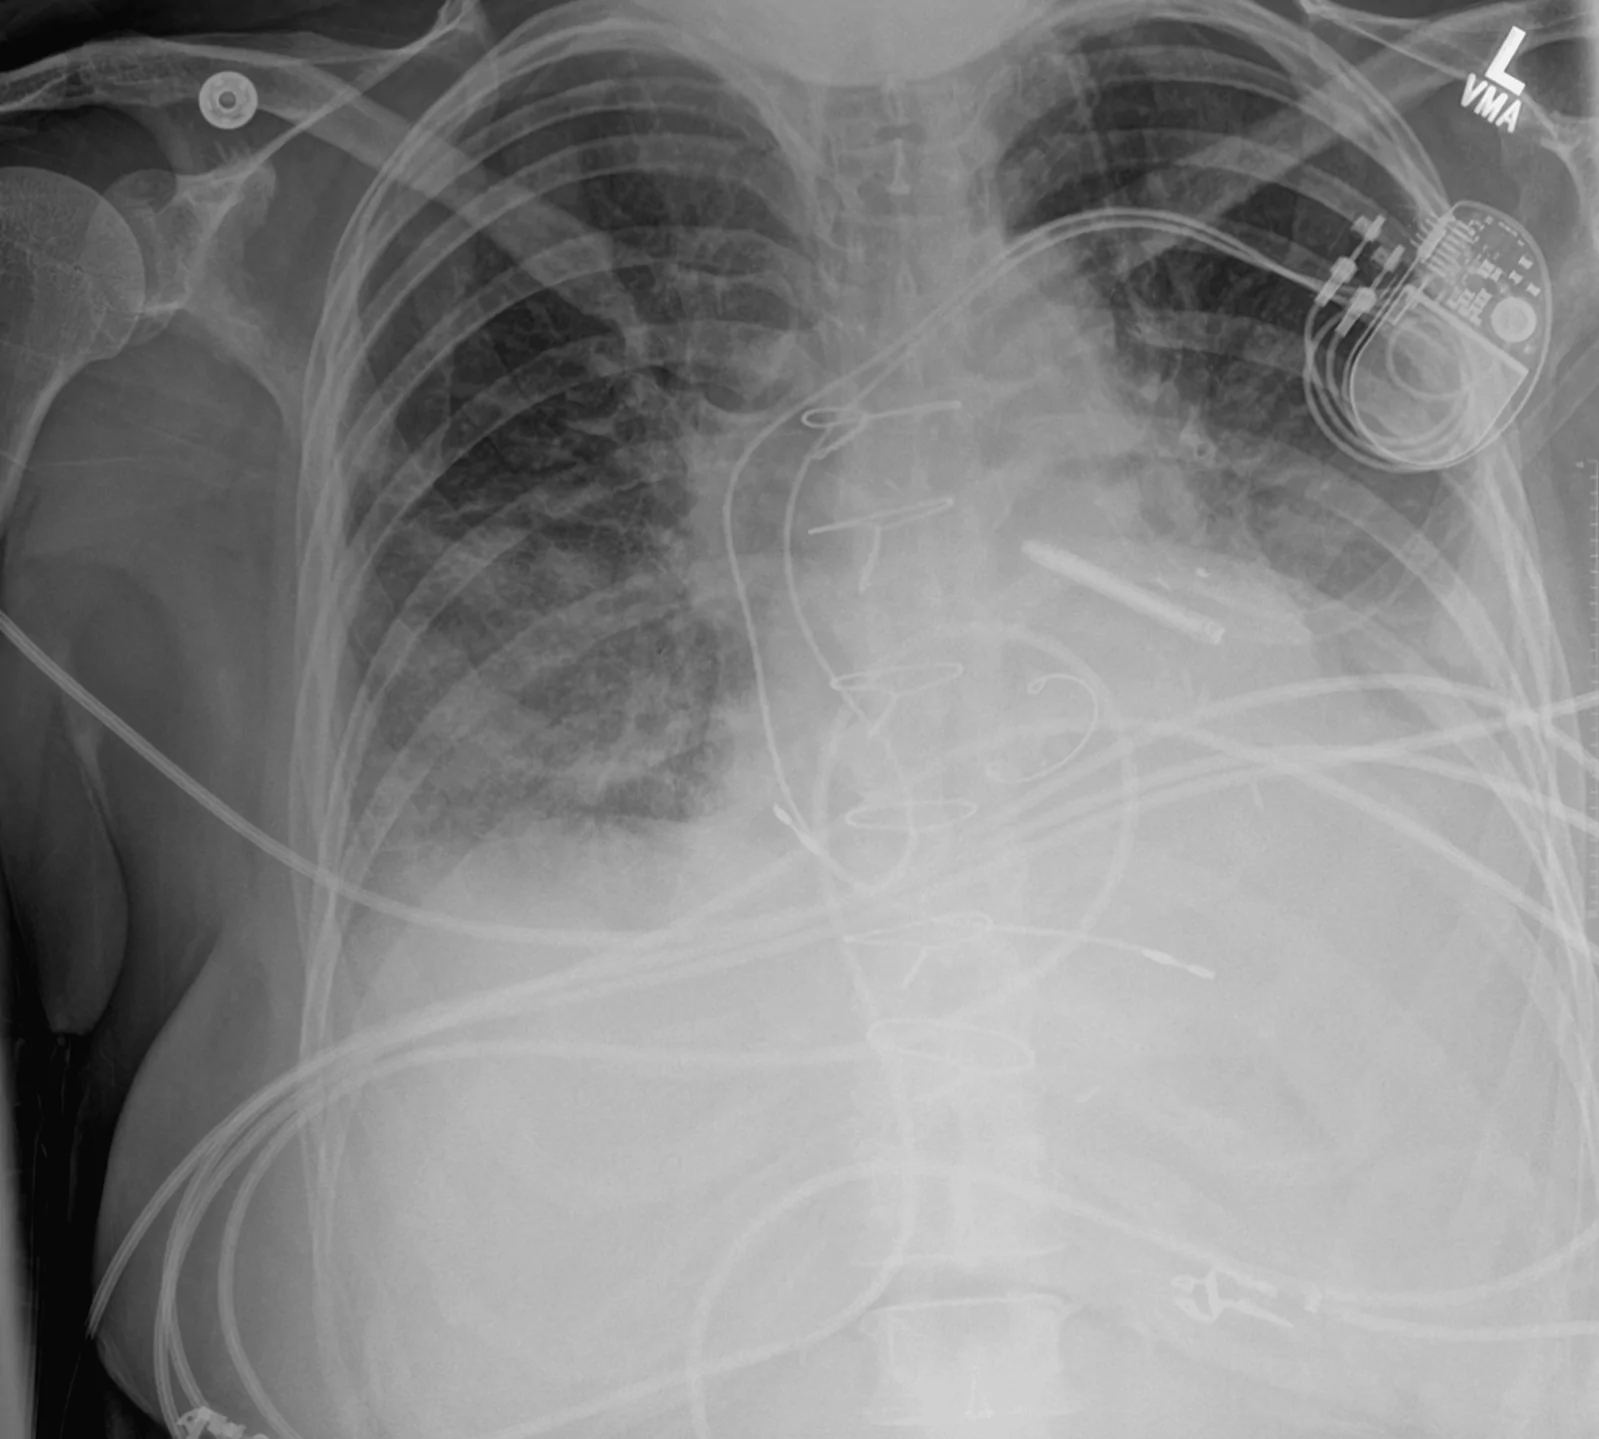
Repeat chest radiograph two hours post-thoracentesis revealing new, prominent bilateral pulmonary infiltrates consistent with suspected REPE following right-sided thoracentesis. REPE, reexpansion pulmonary edema
